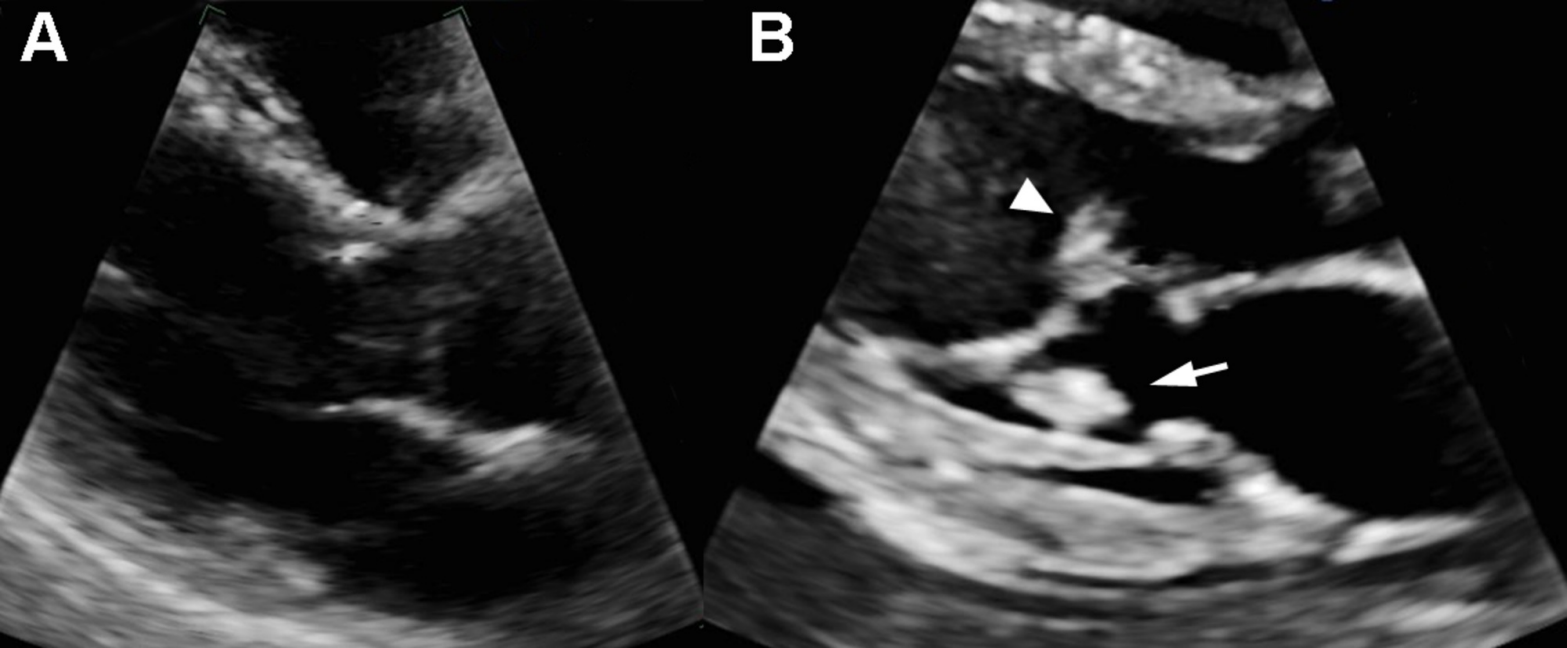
Echocardiogram two weeks prior and on the day of admission Two weeks prior to the current presentation, no obvious vegetations were identified on echocardiogram (A). A repeat echocardiogram at this admission (B) demonstrated two vegetations attached to the anterior leaflet chordae (arrowhead) and the posterior leaflet chordae (arrow) of the mitral valve, respectively.
